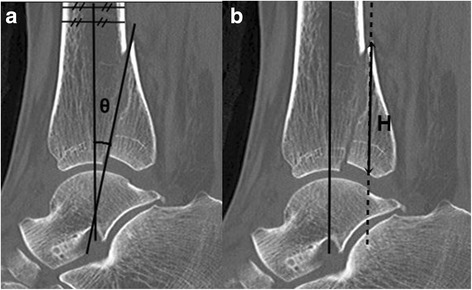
The radiographic measurements on the sagittal reconstructionimages. a The sagittal angle (θ) was measured relative to the neutralaxis and the major fracture line of the posterior fragment on thesagittal reconstruction images. b The largest distance from the apexof the fragment to the point which is crossed by the dotted line andthe articular surface on consecutive sagittal reconstruction views isdefined as the fragment height (FH)
